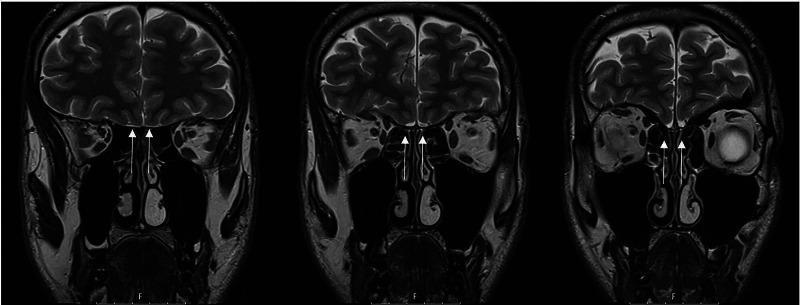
Cerebral and Paranasal Sinuses MRI. Imaging of the paranasal sinuses, olfactory groove and brain confirmed the complete agenesis of both olfactory bulbs (arrows) and did not reveal any other associated nervous or intracranial changes.
